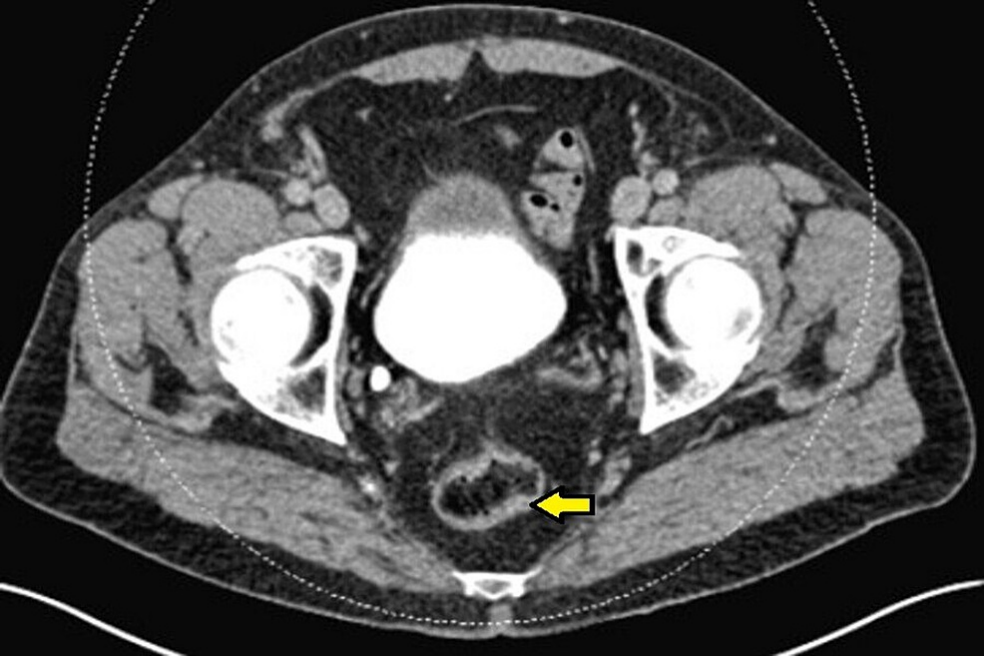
CT of the abdomen and pelvis revealed abnormal thickening of the sigmoid colon (yellow arrow). Initial workup for lower abdominal pain and intermittent hematochezia revealed a thickened rectosigmoid with a broad differential diagnosis. CT: computed tomography
